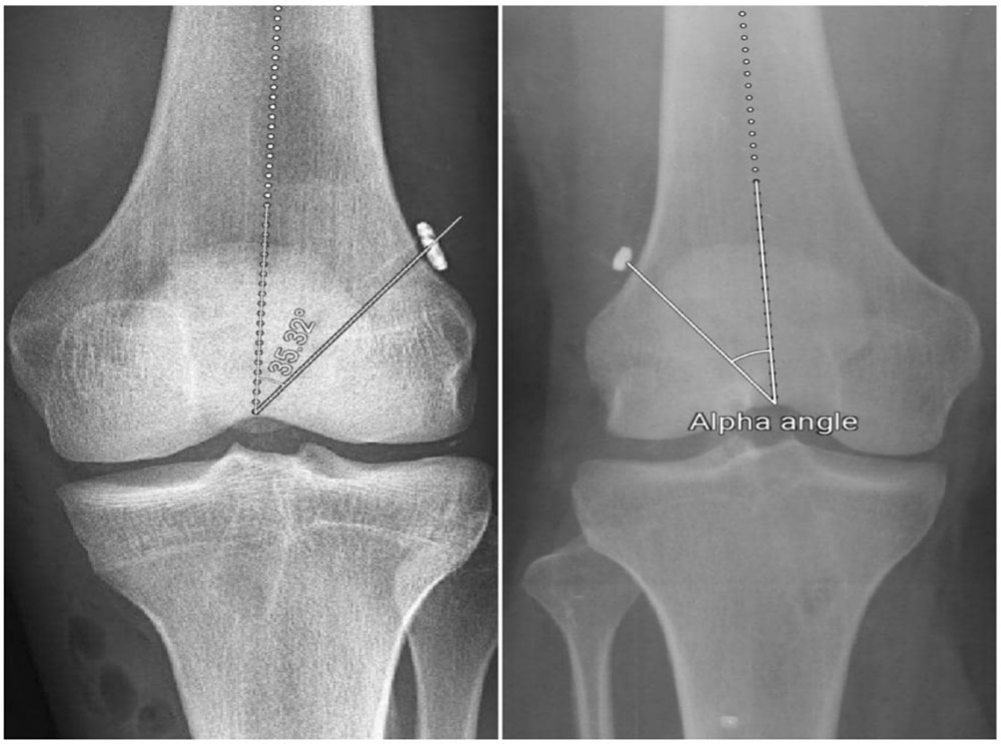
Alpha angle measured in anteroposterior radiographs.
